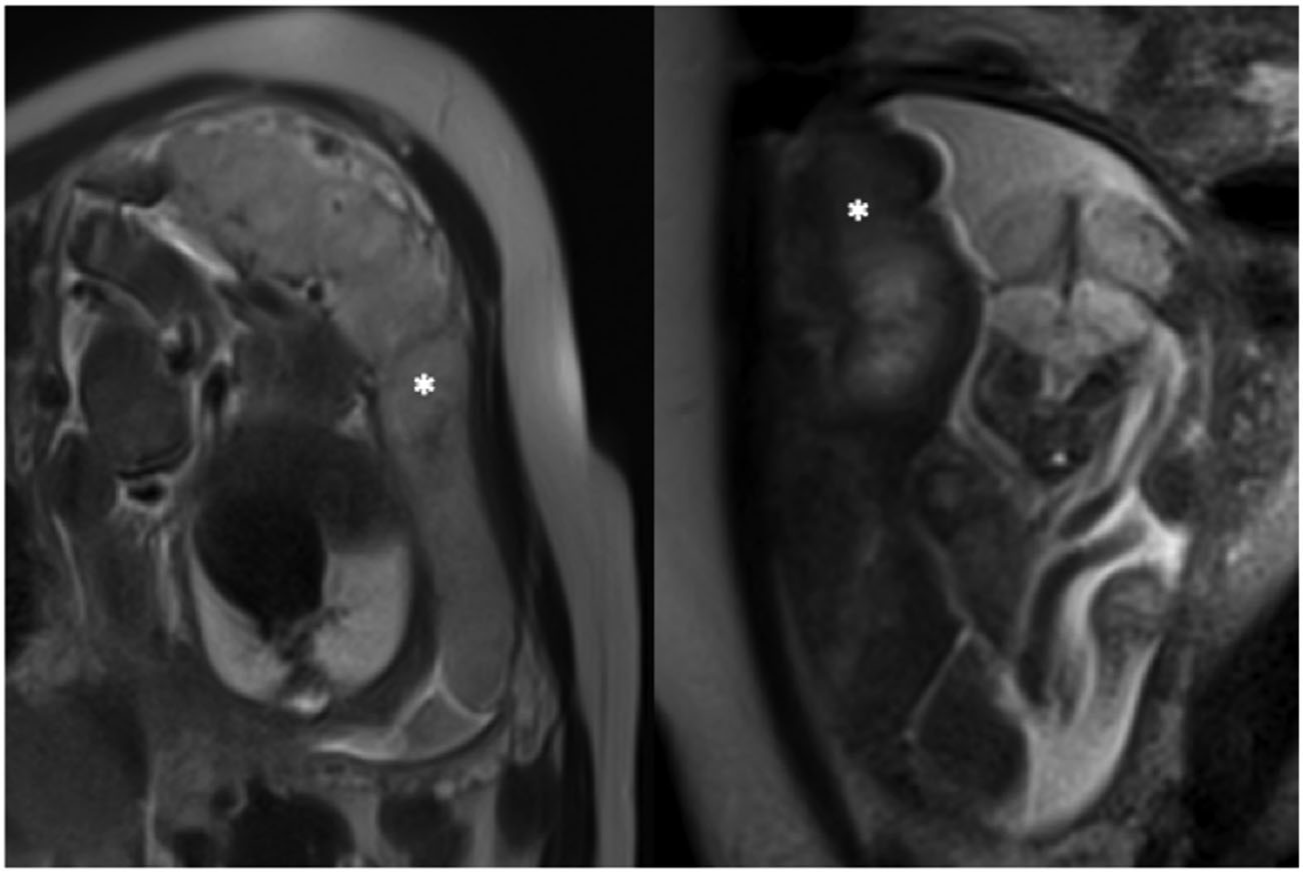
MRI of placenta from a normally grown (left) and FGR (right) fetus. The placenta are marked with white stars. Note the difference in appearance in T2 weighted imaging, with the normal placenta appearing lighter in colour and more homogeneous
